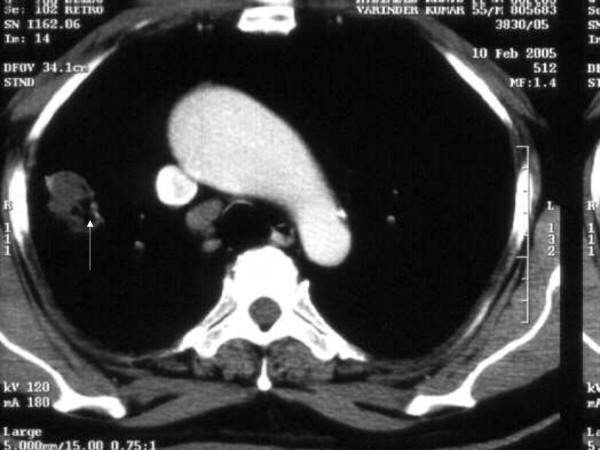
CT scan showing a solitary pulmonary nodule (arrow) with focal areas of cavitation and spiculated margins in right lung.
